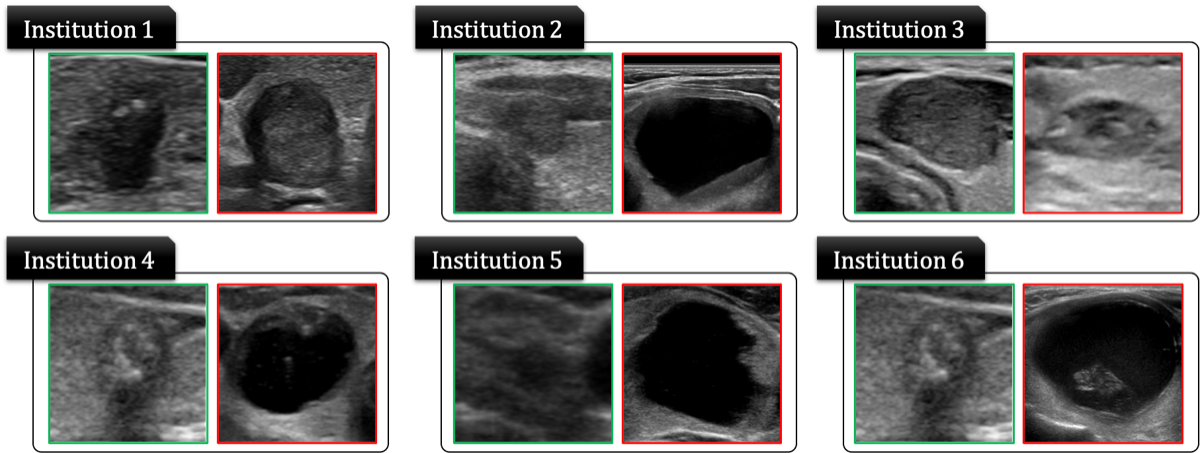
Thyroid ultrasound image data collected from 6 medical institutions to verify federated learning.
